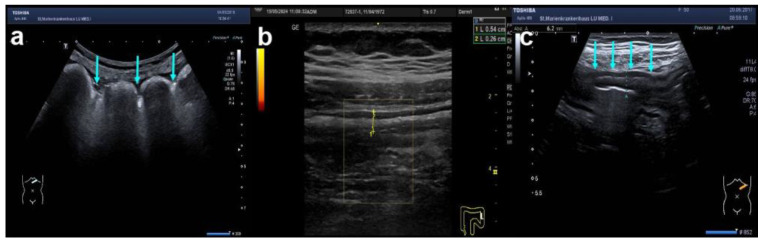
Variants of colonic wall haustration in IBD using B-mode ultrasound. Typical examples are shown as follows: (a) longitudinal section of the left transverse colon showing preserved haustration (turquoise arrows); (b) longitudinal section of the descending colon with increased BWT (yellow markers, number 1), preserved BWS, thickened submucosa (yellow markers, number 2), no hypervascularity (Limberg 1), and complete loss of haustration; (c) longitudinal section of the left transverse colon with increased BWT, thickened mucosa, and reduced haustration (turquoise arrows).
